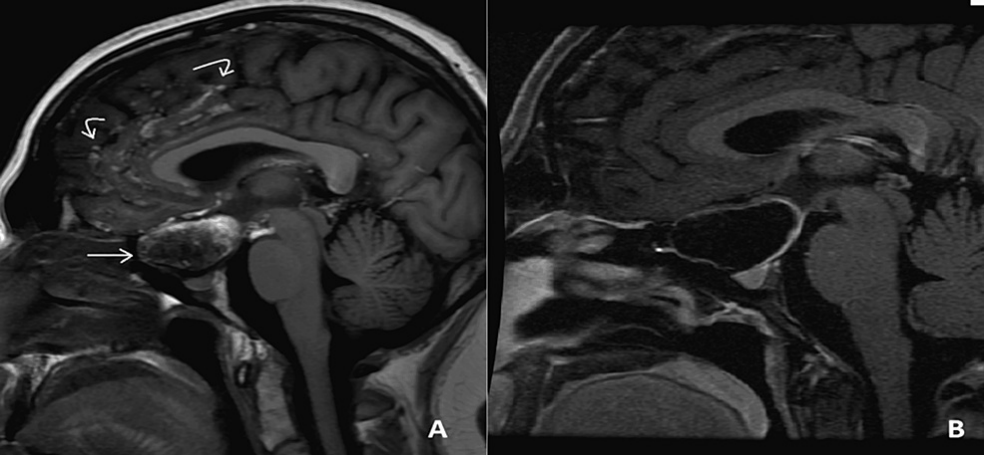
MRI sagittal views of the dermoid cyst (straight arrow) and subarachnoid sulcal cholesterol deposits (curved arrows) on non-contrast T1 (A) and fat-suppressed non-contrast T1 (B) sequences. Again, notice the deposits disappear on fat-suppressed sequencing.
